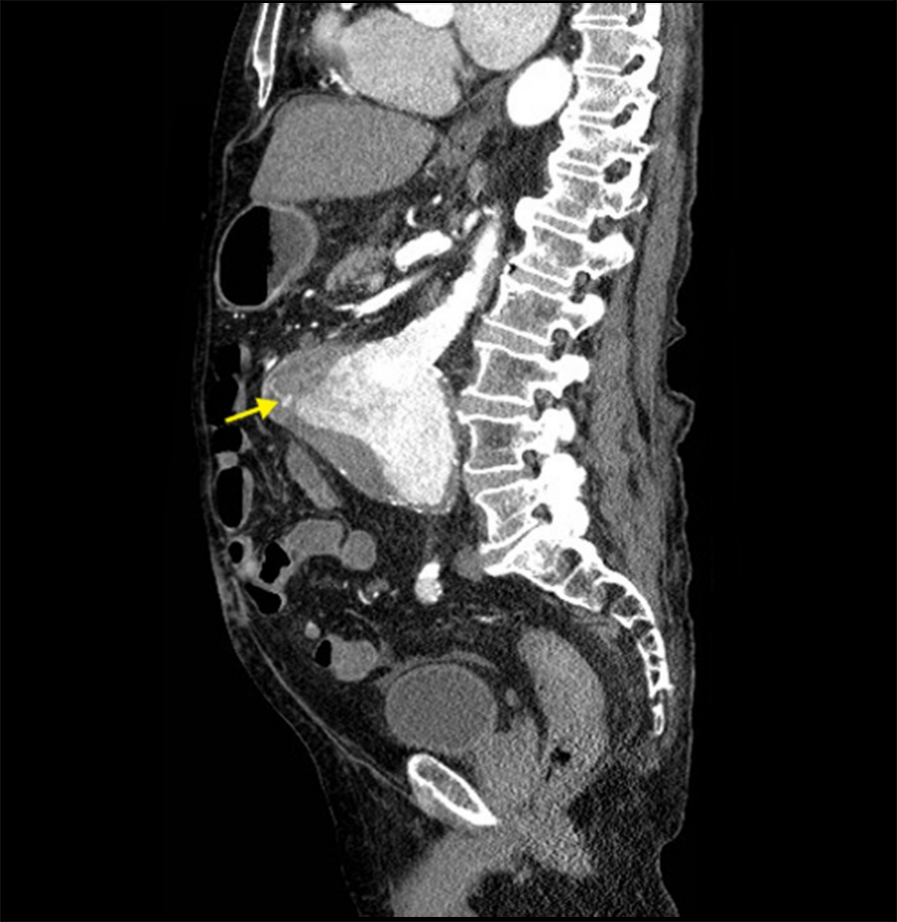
PAEF From Another View Sagittal reformatted contrast-enhanced (IV only) CT image showing the presence of an AAA and associated fistula (arrow).
